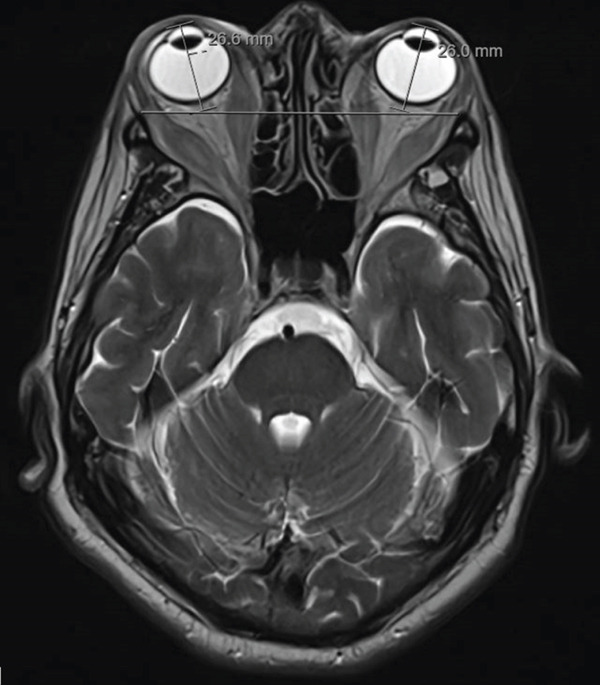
MRI showing inflammatory orbitopathy causing exophthalmos.
